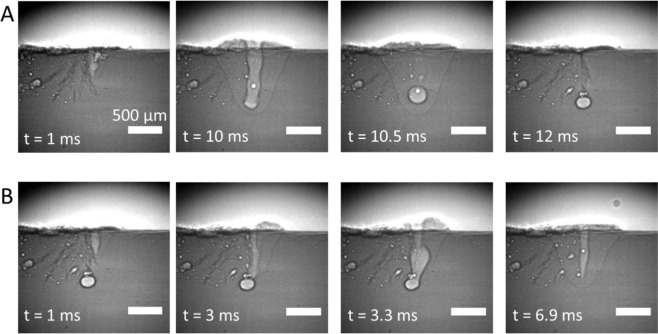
X-ray radiographic images of pore formation (A) and removal (B) during two successive laser pulses. The formation was caused by the rapid collapse of the keyhole channel, leading to vapor being trapped. On the other hand, the removal of the pore was by merging with the keyhole formed by the following laser pulse. Both laser pulses were of 1 kW power and 10 ms pulse duration. The sample was translated relatively to the laser beam and the X-ray beam at a constant velocity of 1.5 mm/s. The timestamp starts simultaneously with the laser illumination. The marker in the right bottom corresponds to a distance of 500 µm.
